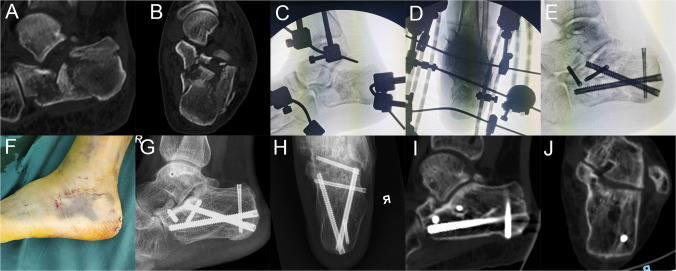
Case example of a Sanders type IV DIACF with an intact tuberosity treated with MIS. (A, B) Preoperative CT scans showing the fracture with an intact tuberosity fragment. (C–F) Intraoperative photographs showing sequential steps: restoration of calcaneal morphology via ligamentotaxis, articular reduction through STA, and percutaneous screw fixation. (G–J) Follow-up radiographs and CT scans at 27 months demonstrating maintained reduction and complete fracture healing. CT, computed tomography; DIACF, displaced intra-articular calcaneal fracture; MIS, minimally invasive surgery; STA, sinus tarsi approach
